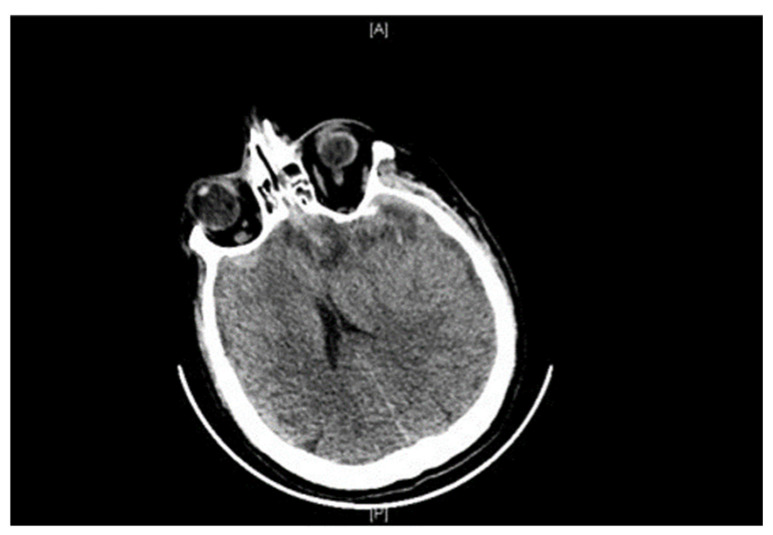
Ante-mortem follow-up CT-scan.
